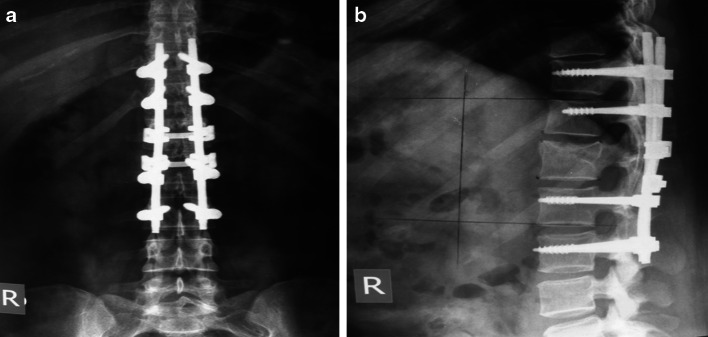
a Postero-anterior and b lateral radiographs of a patient with a T12 vertebra fracture who received LS instrumentation
